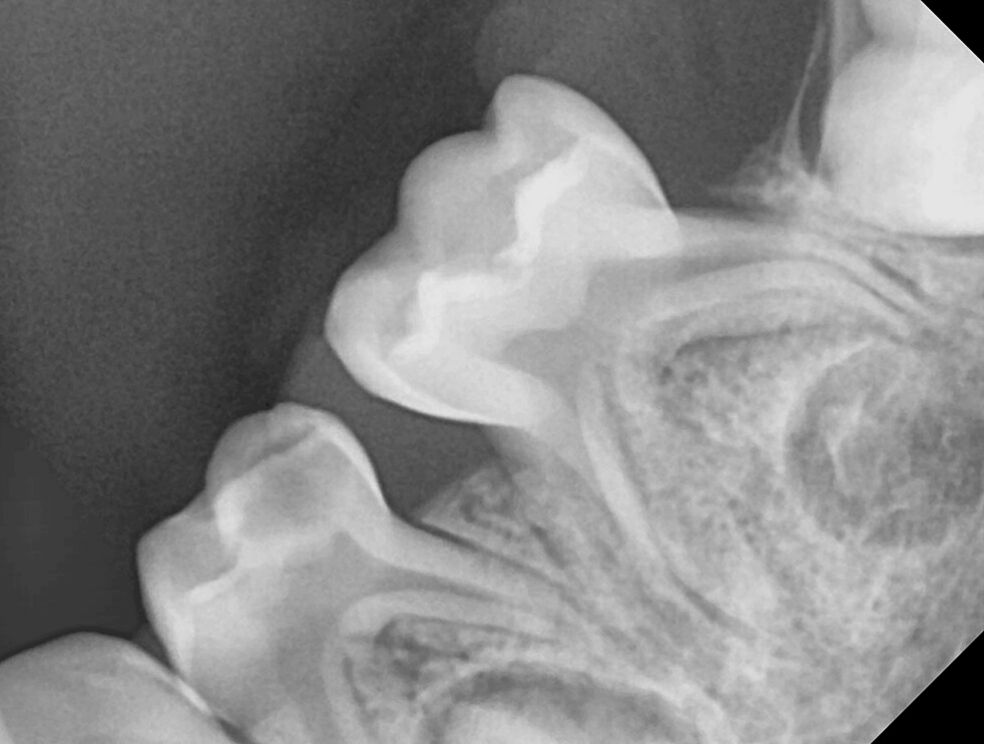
Radiographic image of the involved area
